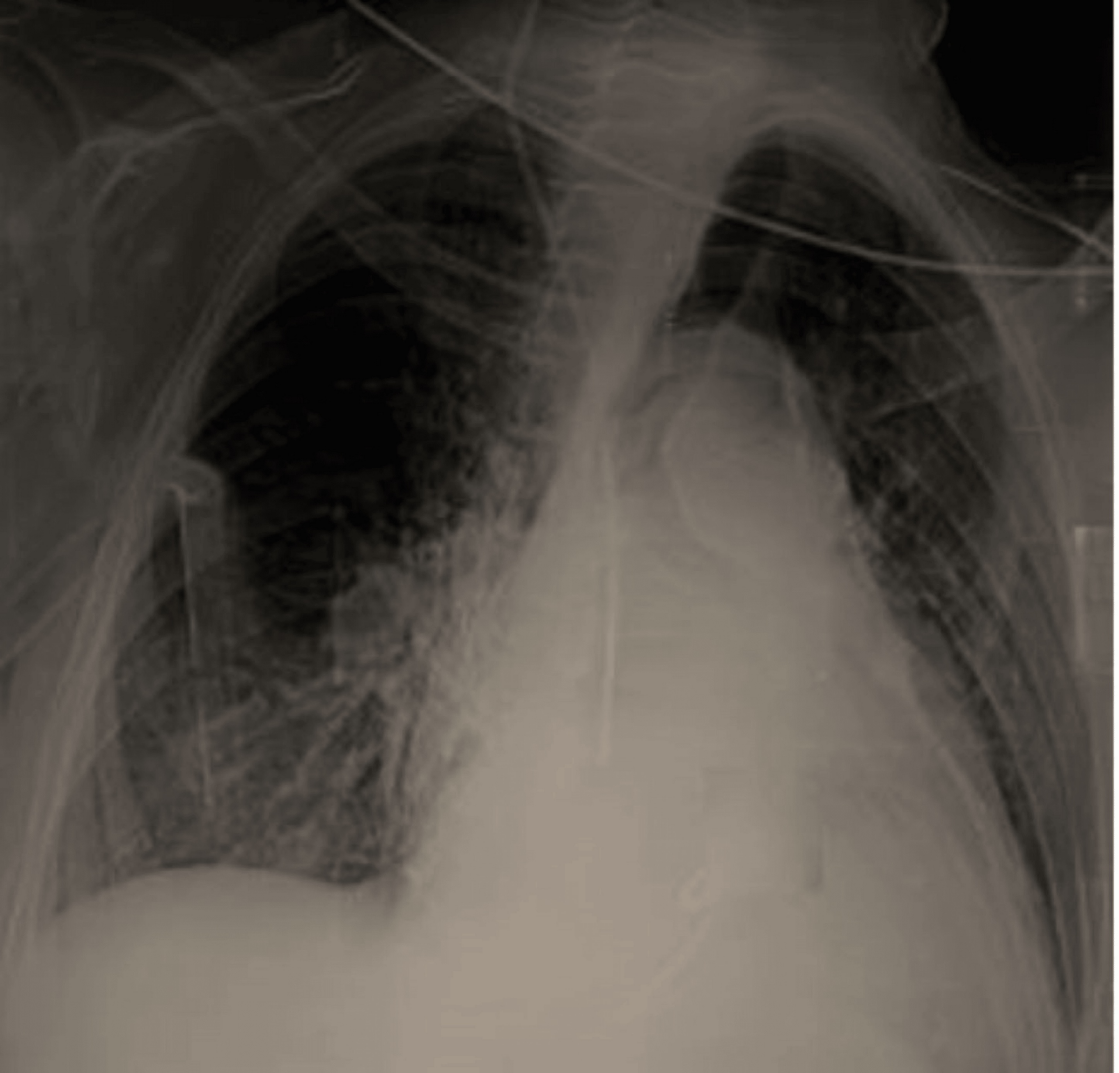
AP chest X-ray of the same patient after drainage, showing a complete resolution of the effusion. AP, anteroposterior
